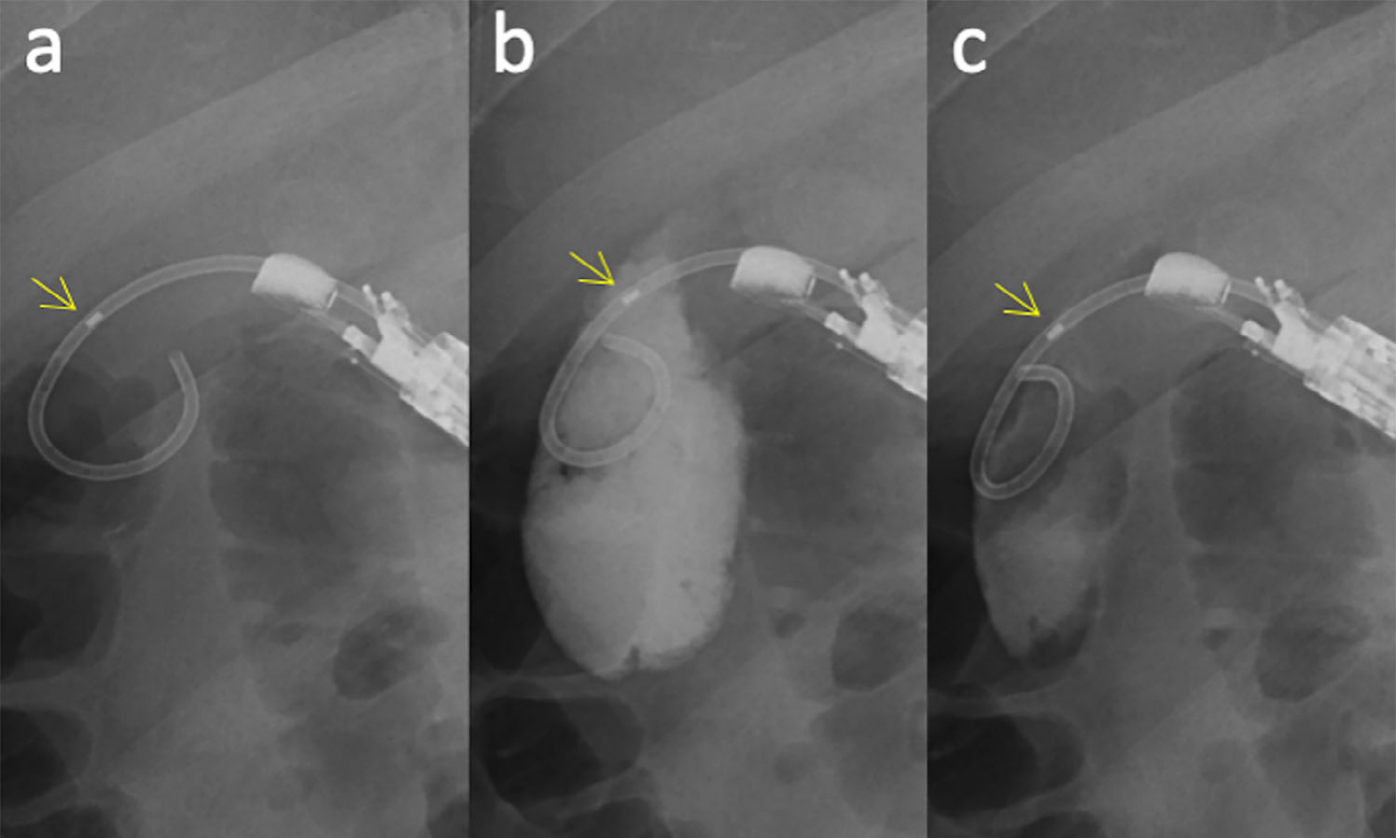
Gallbladder lavage using a DPPS delivery system during EUS‐GBD. (a) After removing a guidewire, the inner sheath is retracted to the halfway‐withdrawal position within the DPPS, and multiple holes emerge in the distal pigtailed area. At this position, the gallbladder contents are sufficiently aspirated from the inner sheath. (b) Contrast‐containing saline is injected via the inner sheath. (c) The next aspiration. The lavage is repeated until the aspirated bile changes into serous. The yellow arrows indicate the radiopaque marker at the tip of the inner sheath. DPPS, double‐pigtail plastic stent; EUS‐GBD, endoscopic ultrasound‐guided gallbladder drainage.
